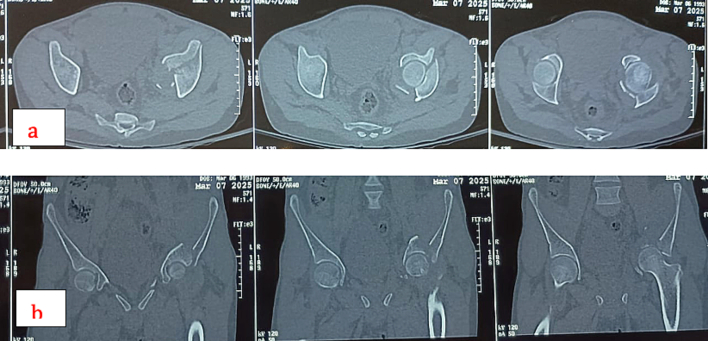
(a) Preoperative CT scan [axial sections]. (b) Preoperative CT scan [coronal sections].
